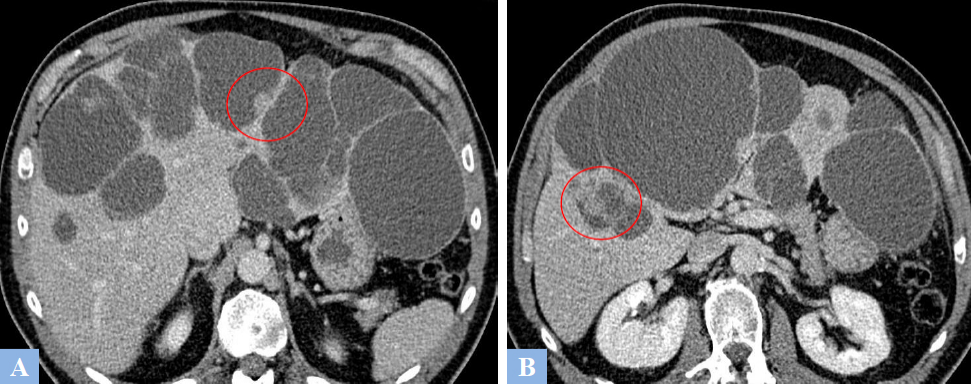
Cystic metastases from a rectal neuroendocrine tumor discovered in a 54-year-old patient presenting with right hypochondrium pain. A. CT image in the axial plane at the portal phase of enhancement. Multiple hepatic cystic lesions with intracystic vegetations (red circle) are consistent with secondary lesions. B. Same study, lower slices. Multiple hepatic cystic secondary lesions with intracystic vegetations (red circle).
